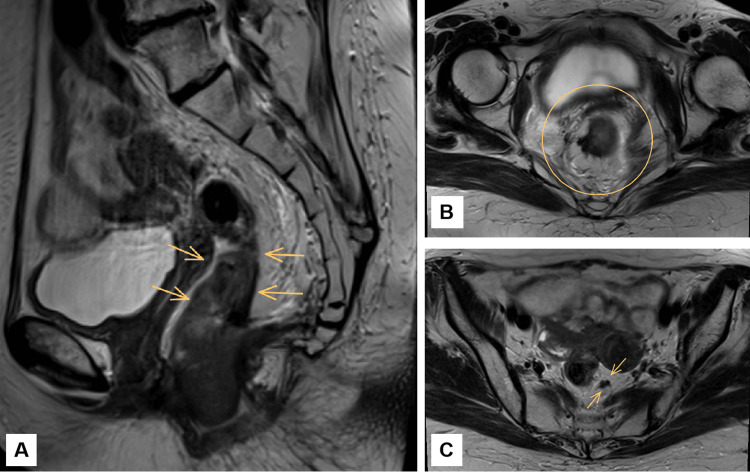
Latest pelvic MRI demonstrating post-treatment findings after total chemoradiotherapy. A. Sagittal T2-weighted pelvic MRI demonstrating uniform hypointense thickening of the rectal wall at the site of the primary tumor (indicated by arrows), consistent with post-treatment fibrosis. No discrete mass or intermediate signal intensity lesion is seen. The mesorectal fat remains preserved, with no evidence of extramural invasion. These findings are indicative of a complete radiologic response. B. Axial T2-weighted pelvic MRI demonstrating concentric thickening of the rectal wall with low signal intensity in the circled area, consistent with post-treatment fibrosis. The mesorectal fascia is preserved, with no evidence of suspicious mesorectal lymphadenopathy. C. Axial T2-weighted pelvic MRI showing fibrotic thickening at the site of the primary tumor (arrows), with no evidence of residual tumor.
